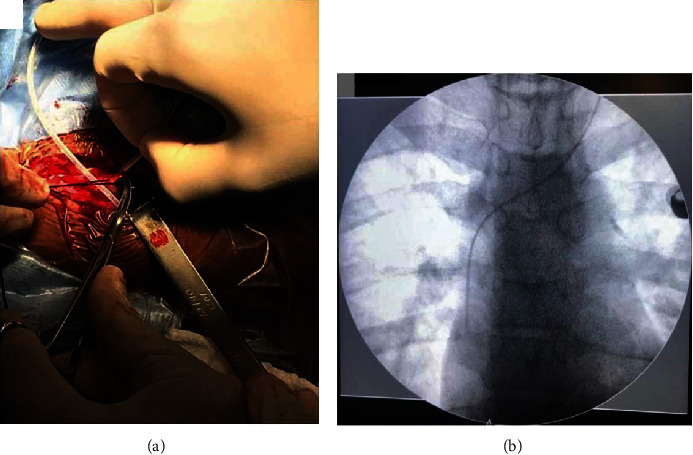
Distal catheter insertion. (a) Insertion of the distal catheter in the right atrium through the left linguofacial trunk. (b) Chest X-ray confirming atrial catheter position.
